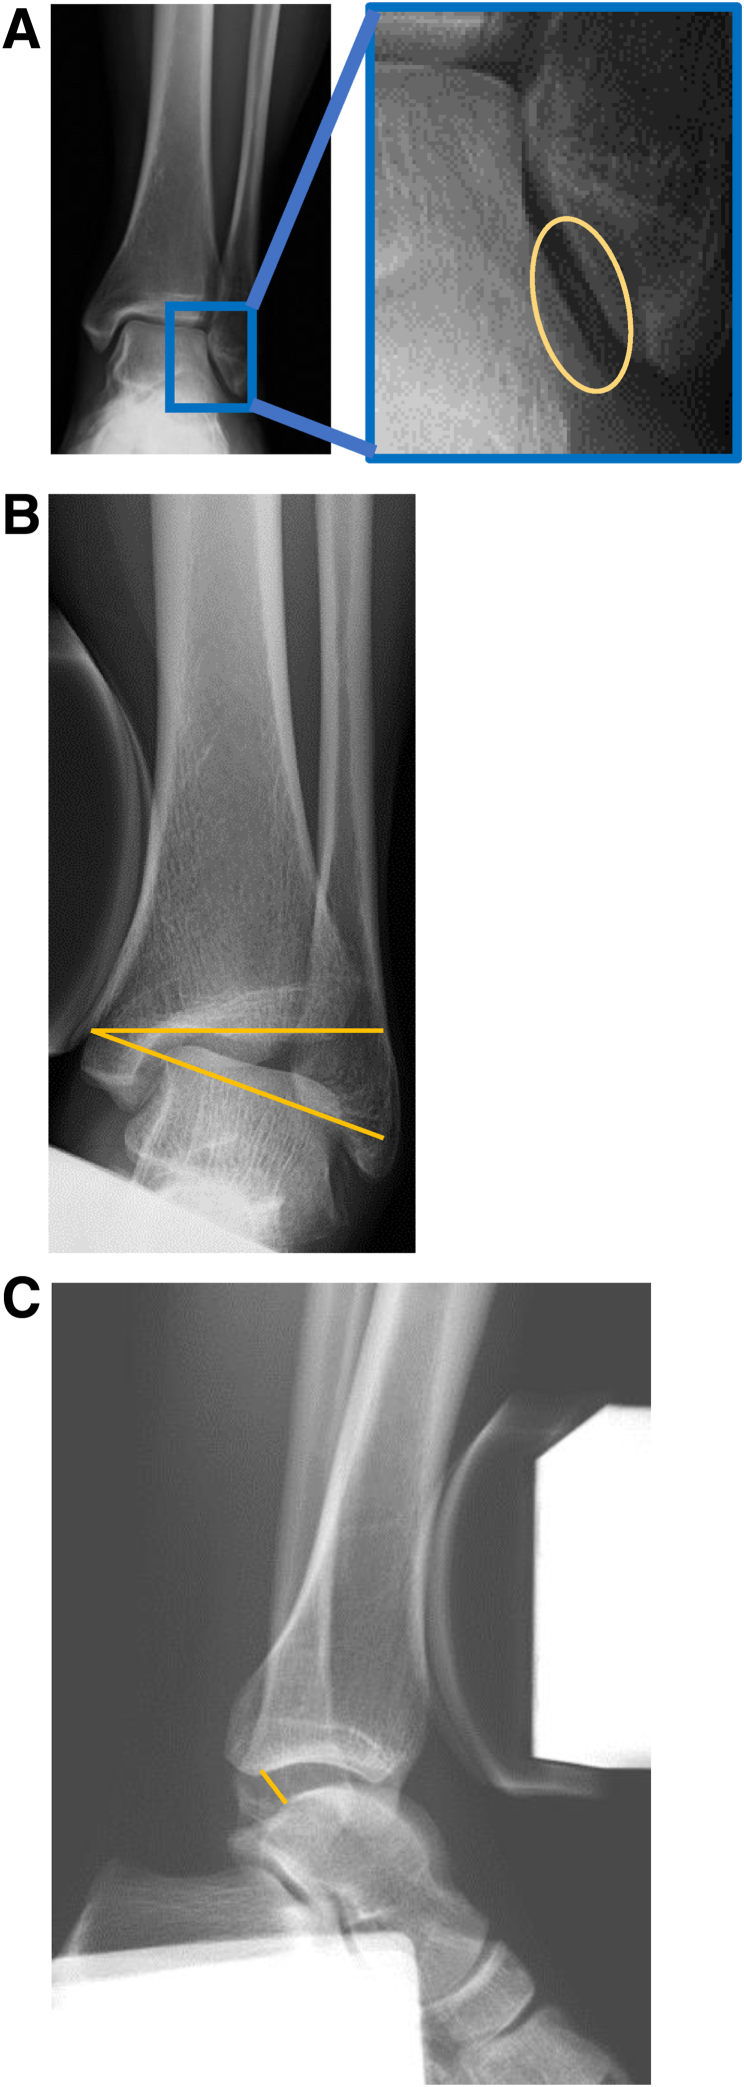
Preoperative radiographic images of left ankle: anteroposterior view. (A) A thin bone fragment is observed between the talus and fibula (oval). (B, C) Stress radiographs are obtained using a Telos stress device with a magnitude of 150 N. The inversion stress test shows an increase in the talar tilt angle (lines) (B), and the anterior drawer test shows an increase in the anterior drawer distance (line) (C).
